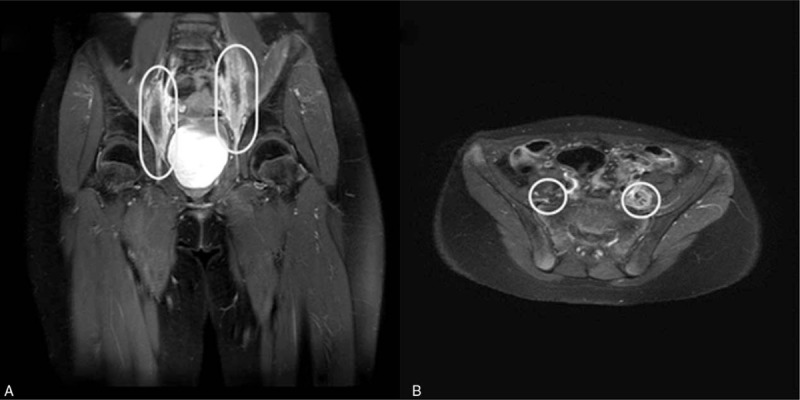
Magnetic resonance imaging scans. (A) Coronal image and (B) axial image.
